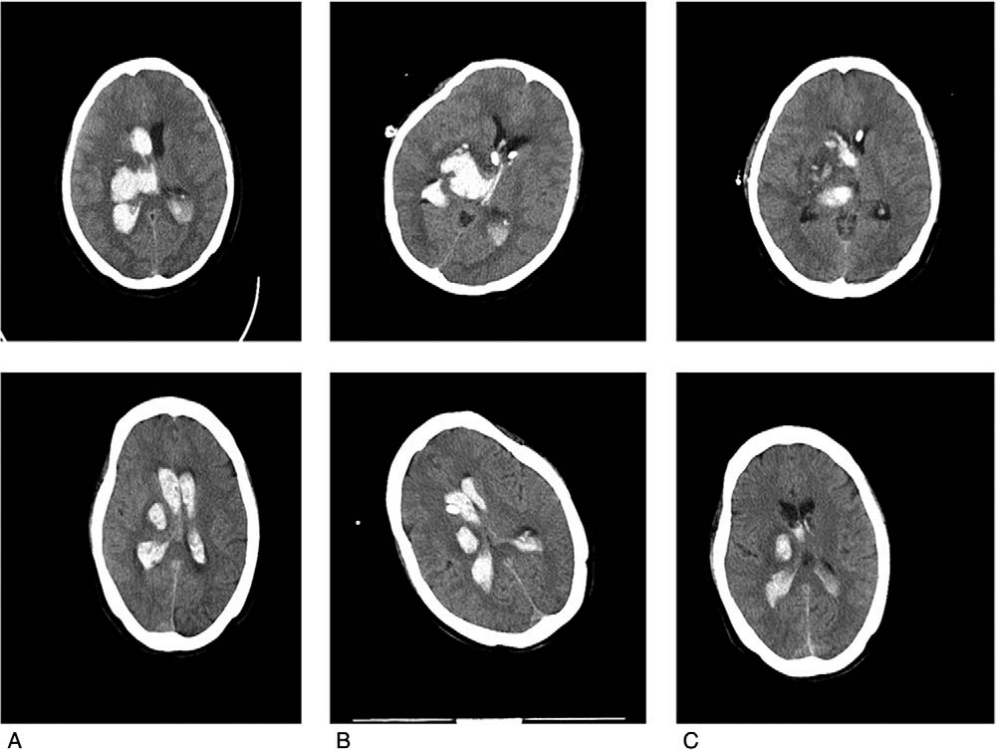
Pre- and postoperative CT scan images of severe IVH treated by EVD. (A) CT scan image at onset, with IVH and ventricular enlargement. (B) CT scan image 12 h after the EVD; most hematoma was not cleared. (C) CT scan images in the first week after the surgery. Most hematoma was absorbed, and the tube was still placed in the ventricle. CT, computed tomography, EVD = extraventricular drainage, IVH = intraventricular hemorrhage.
